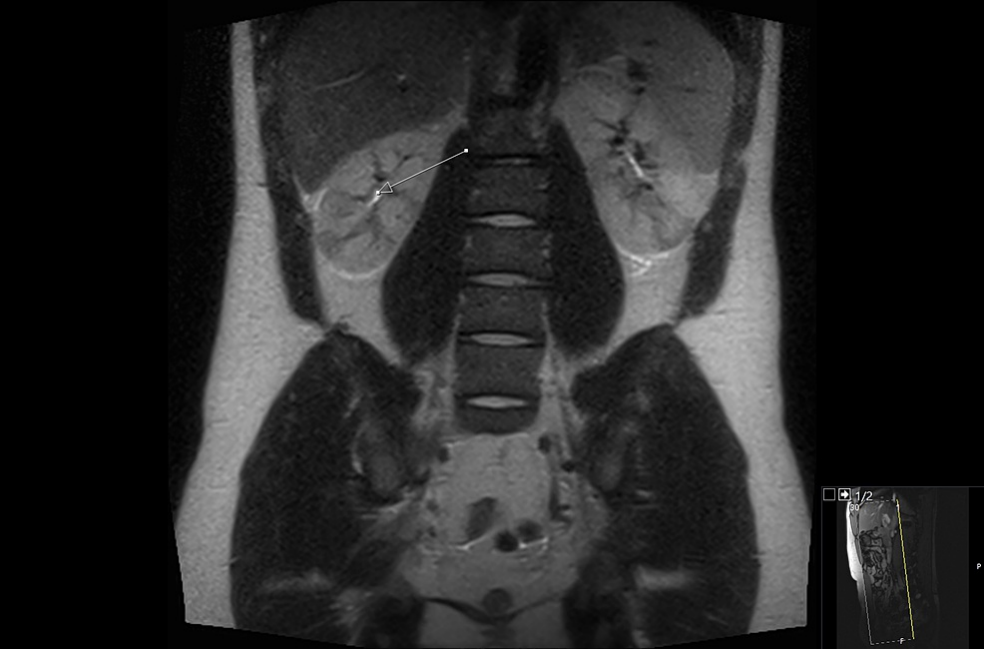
MRI AP showing the T2 acquisition coronal/axial wedge like areas of relative hypo-intense changes in the kidneys, as shown by the pointed arrow.
